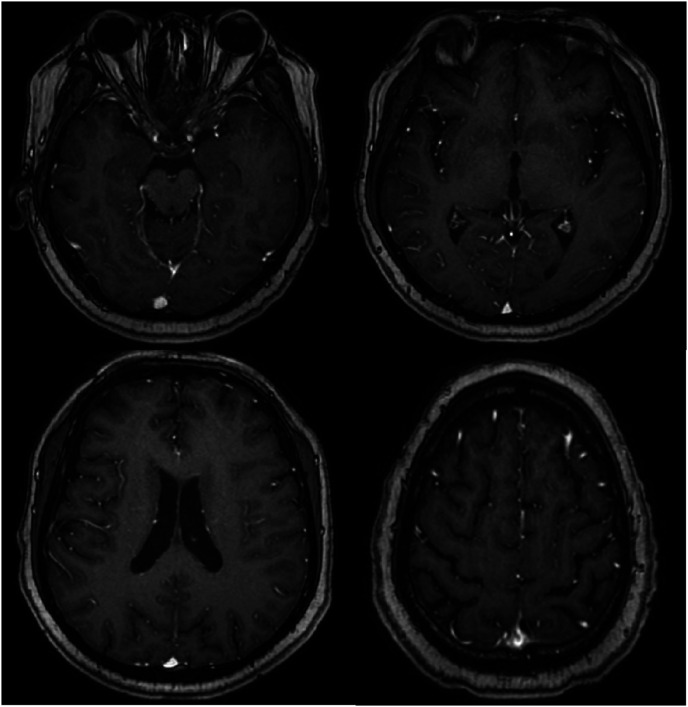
Magnetic resonance imaging of the brain, T1-weighted sequence, showing no significant abnormalities.
